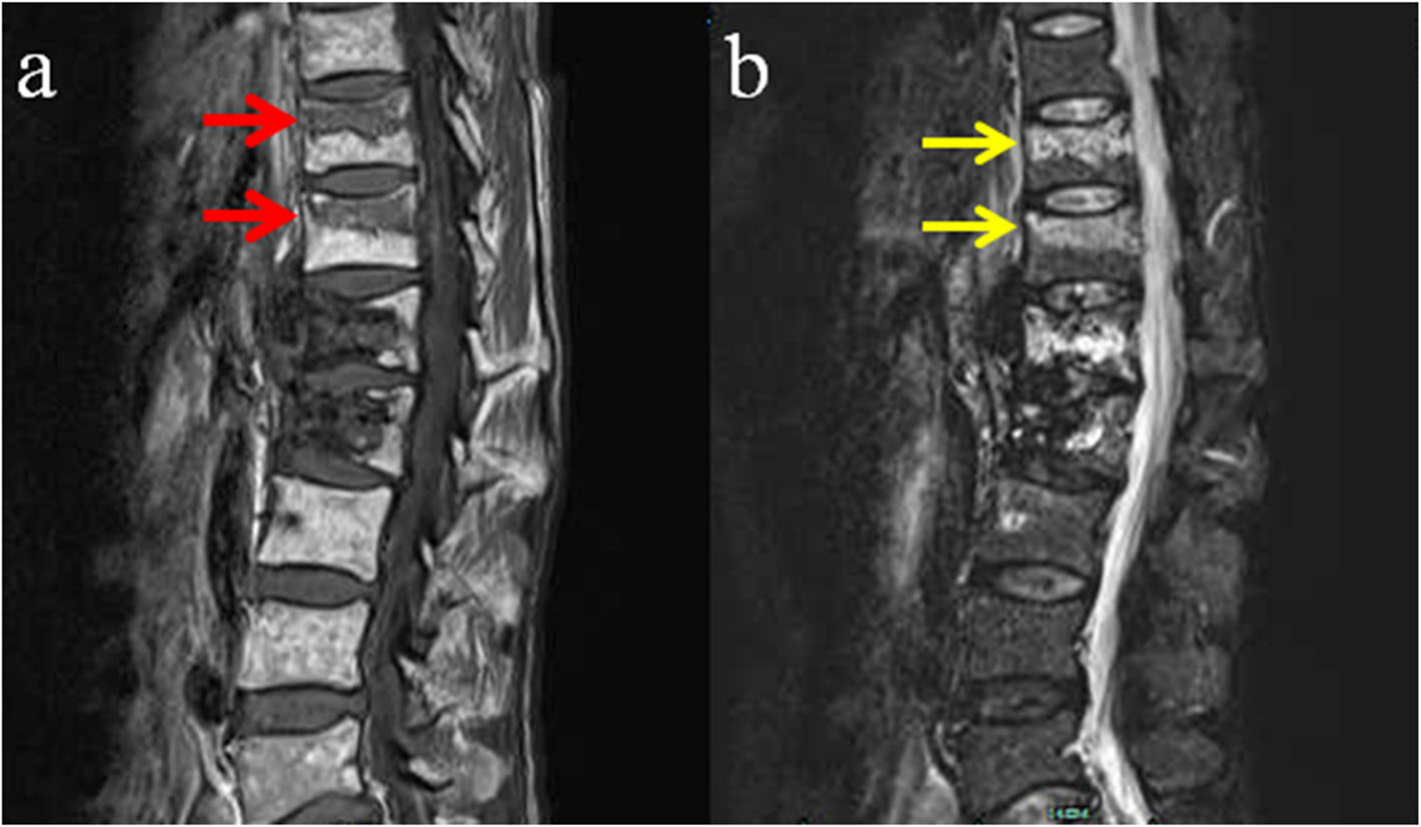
MRI images at 9 years after radiotherapy following trauma: a T1WI demonstrating hypointense signal changes of the T11 and T12 vertebrae (red arrow). b T2WI showing hyperintense signal changes of the T11 and T12 vertebrae (yellow arrow)
